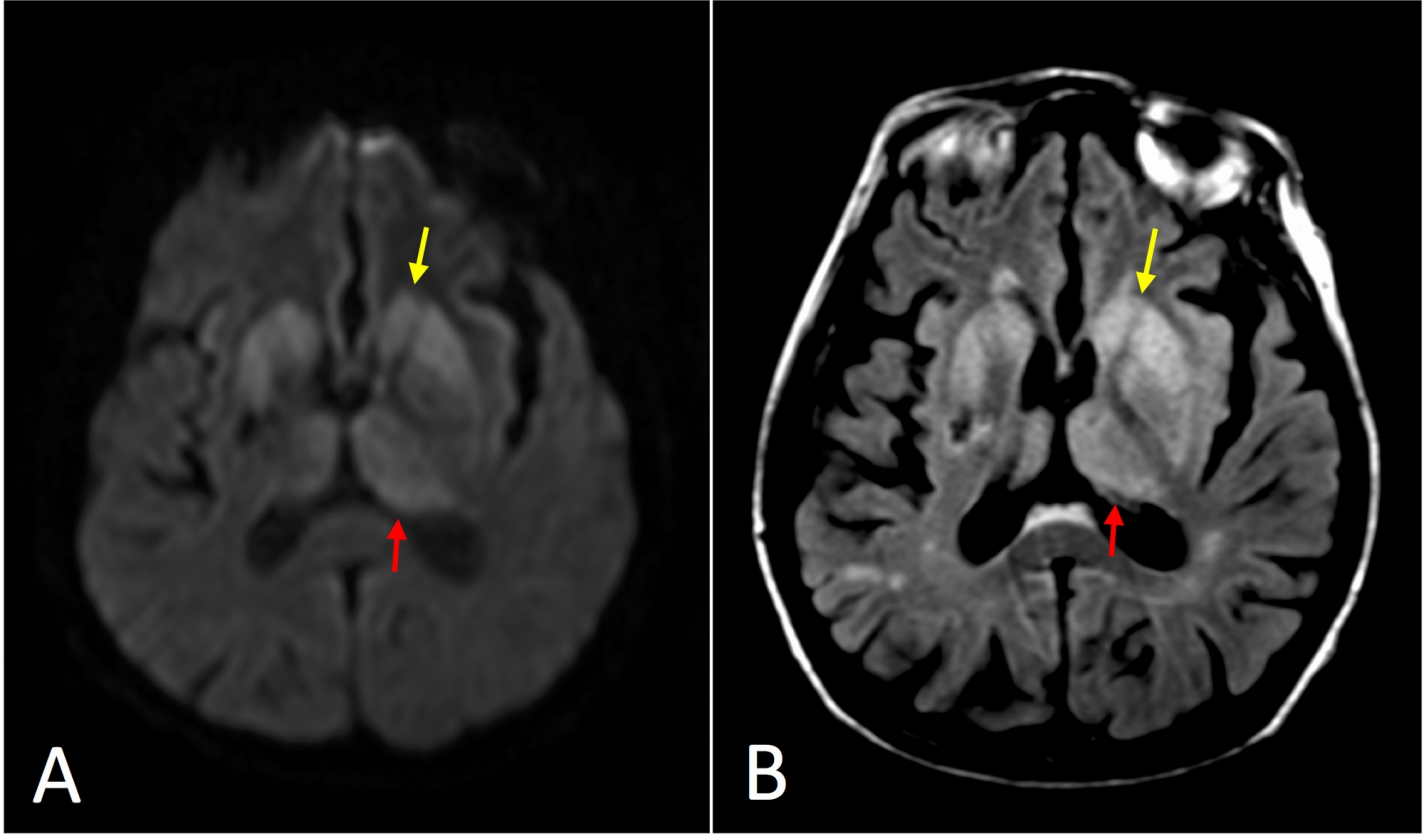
Axial magnetic resonance imaging (MRI) of Case 2. (A) Diffusion-weighted image (DWI) at the level of the basal ganglia demonstrating asymmetrically increased signal primarily in the striatum (yellow arrow) and medial and posterior thalamus (red arrow) reminiscent of the “hockey stick” sign frequently seen in variant Creutzfeldt-Jakob disease (CJD). (B) T2-weighted fluid attenuation inversion recovery (T2-FLAIR) image with less corresponding hyperintense signal.
